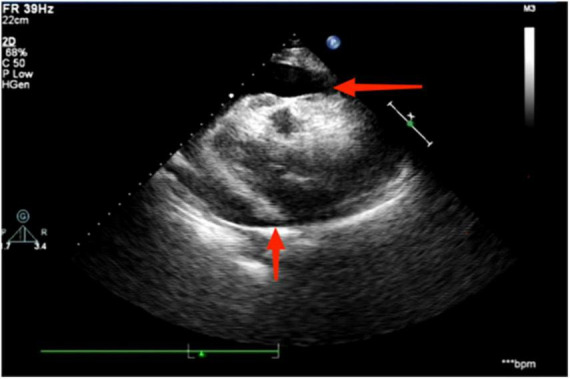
Echocardiography suggests the presence of a moderate to large pericardial effusion, as indicated by the red arrowhead.
